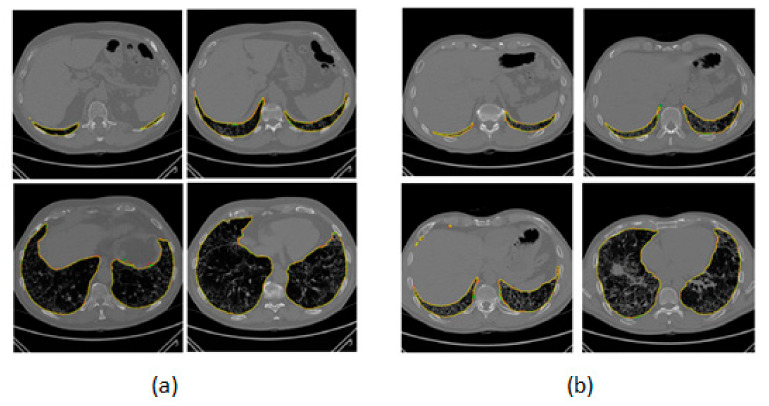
Two examples of lung segmentations (4 slices for both (a,b) high-resolution computed tomography (HRCT) studies). The manual segmentation (yellow), E-Net (red), and U-Net (green) are superimposed in both (a,b) images.
